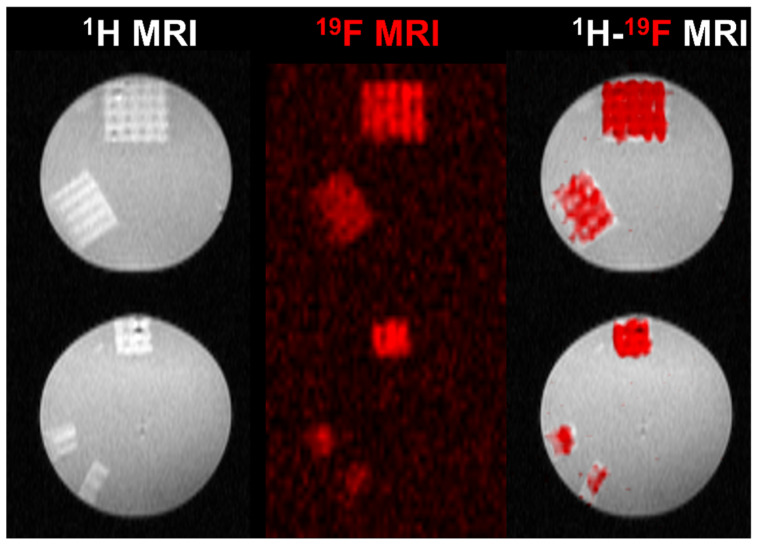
^1^H (gray scale), ^19^F (red scale), and dual ^1^H-^19^F MRI of prepared 3D-printed scaffolds reswollen in PBS (140 mM, pH 7.4) measured at 30 °C. The scaffold mass was (top to bottom) 76.6, 38.3, 19.1, 9.5, and 4.7 mg.
